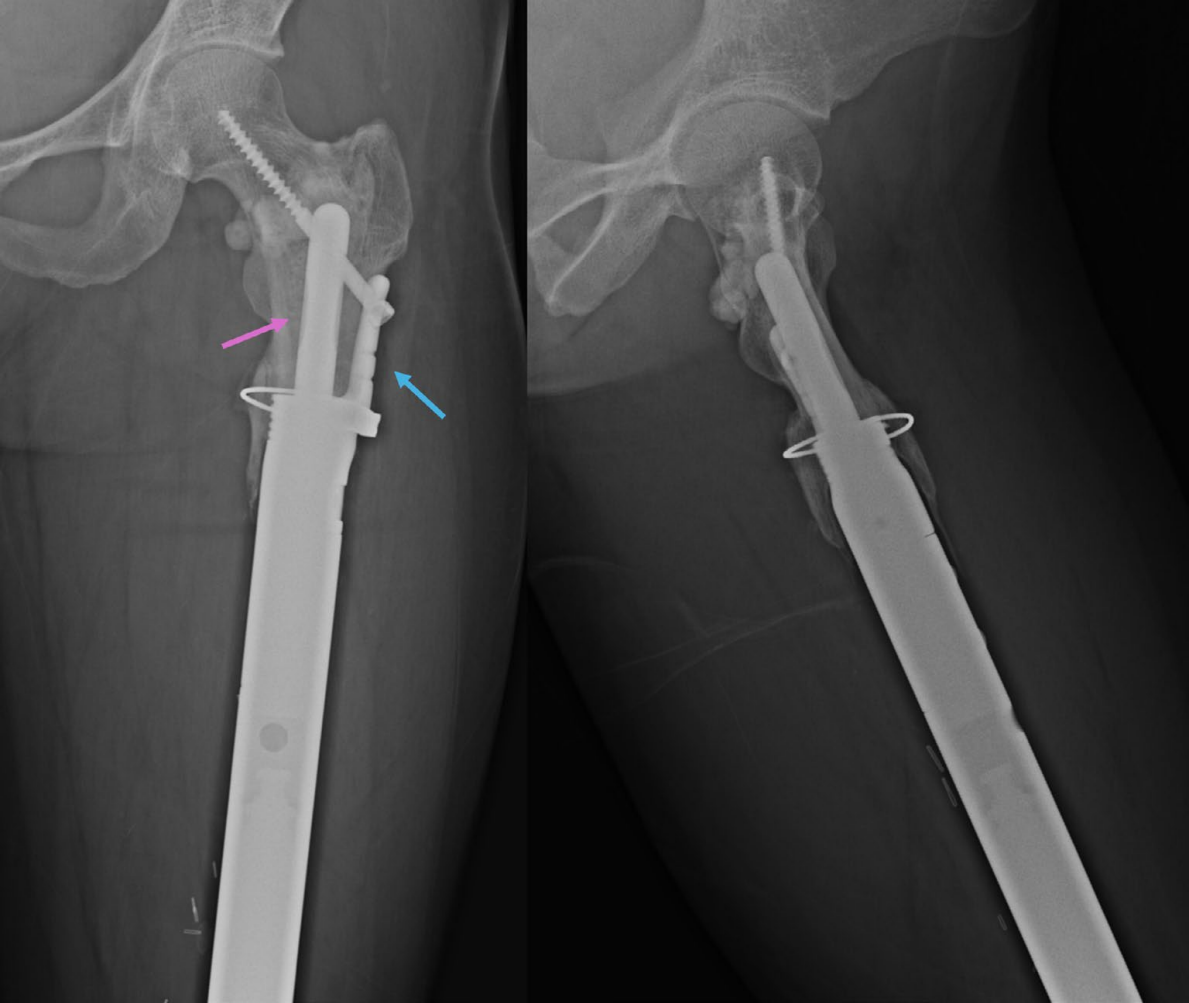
Plain radiographs of a distal femur endoprosthesis with a HA‐coated stem collar, short‐segment cemented stem (purple arrow), extracortical plate (blue arrow) and screw fixation into the femoral head. The stem length is 54 mm, and the plate length is 36 mm.
